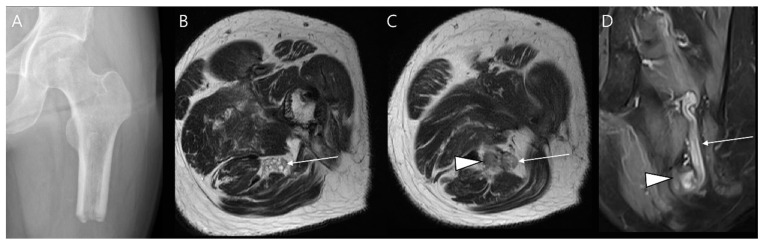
Amputation neuroma. (A) A 48-year-old man with a history of osteosarcoma who underwent an above-the-knee amputation. (B,C) Axial T2-weighted MR images obtained 1 year post-surgery reveal enlargement of the fascicles along the sciatic nerve (arrows). A bulbous mass is visible at the end of the sciatic nerve (arrowhead), suggesting an amputation neuroma. (D) Coronal T1-weighted fat-suppressed contrast-enhanced MR image shows an ovoid mass with minimal enhancement (arrowhead) at the end of the sciatic nerve (arrow).
